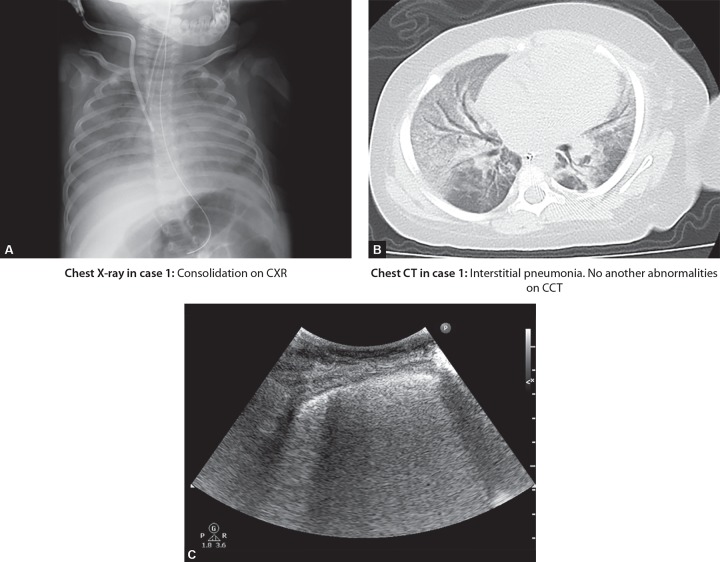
Comparison between chest X-ray, chest CT and lung ultrasound in case 1.
